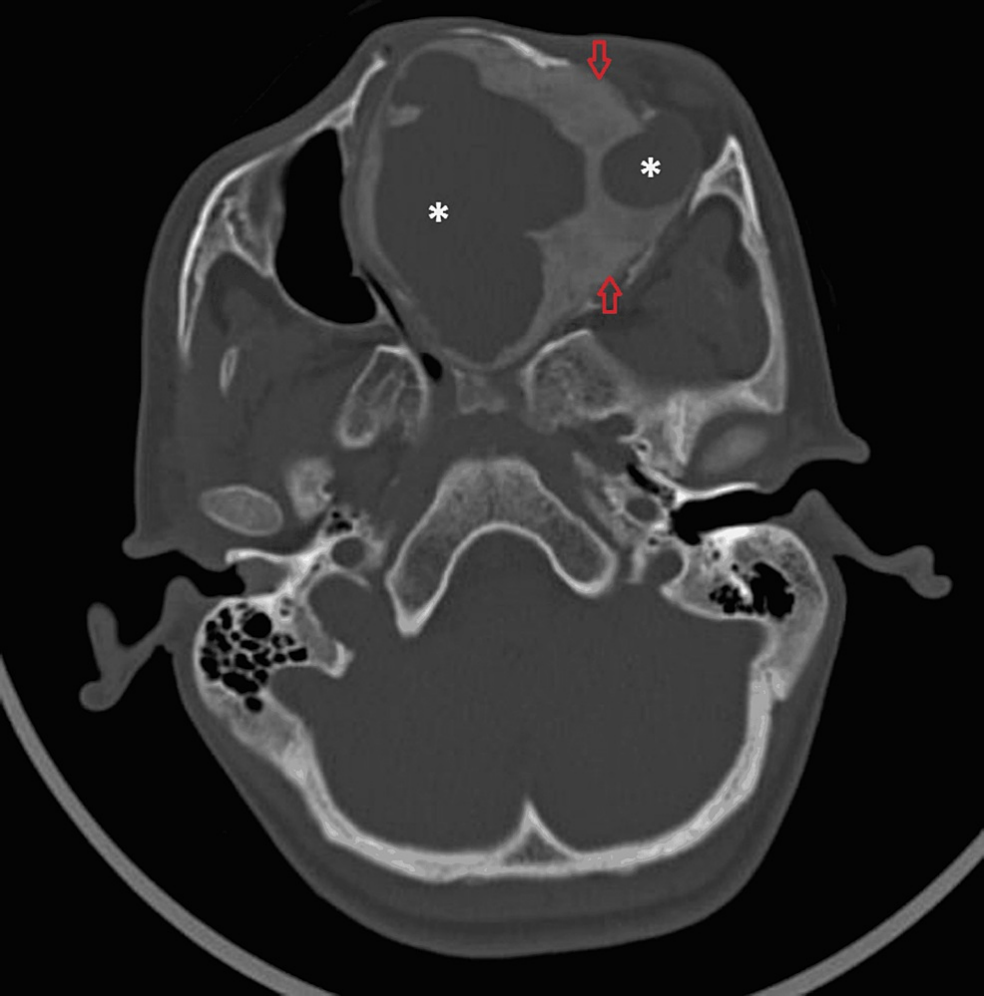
Axial CT image in bone window A lytic expansile lesion involving the nasal cavity central cystic region (white asterisk) and peripheral bone showing ground glass matrix (open red arrows). CT - Computed Tomography
